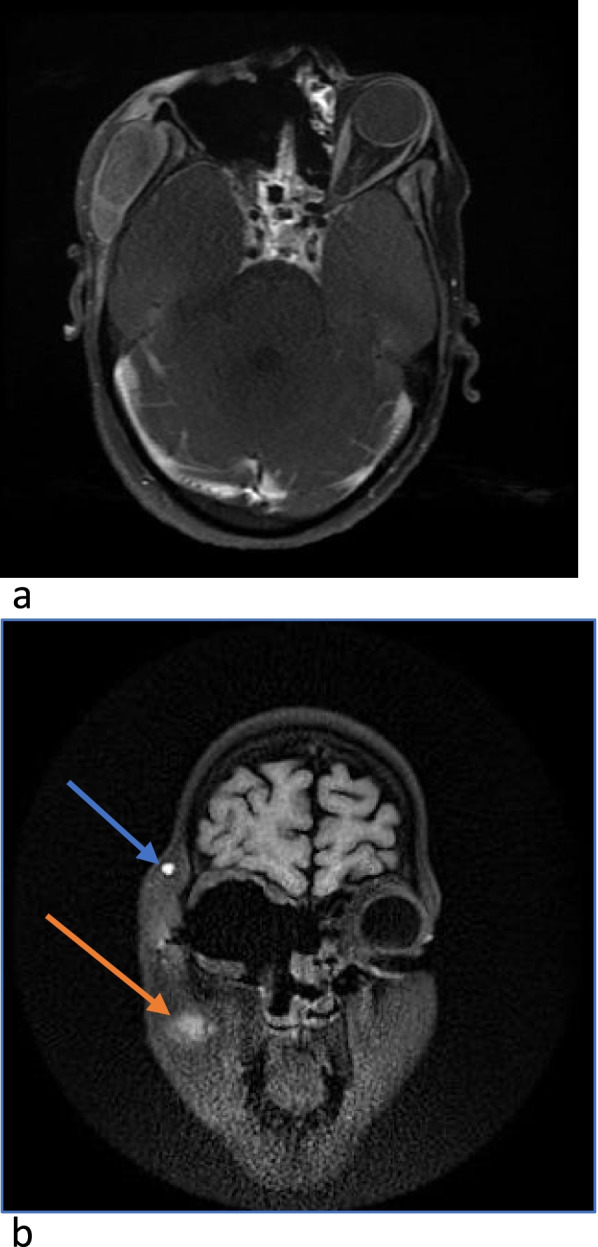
a, b Post-operative MRI shows right maxillary sinus widening with absent right eye globe and intraocular and extraocular muscles along with removed floor of orbit and absent nasal turbinates on the right side. Right orbit is seen communicating with sphenoid sinus on the right side. Fat suppressed hyperintensity seen in the right temporal fossa(blue arrow) and masticator space(red arrow) involving the right masseter and temporalis muscle. Hyperintensity is seen in the floor of the right maxillary sinus showing peripheral and septal enhancement on post contrast. Bilateral cavernous sinus are patent
